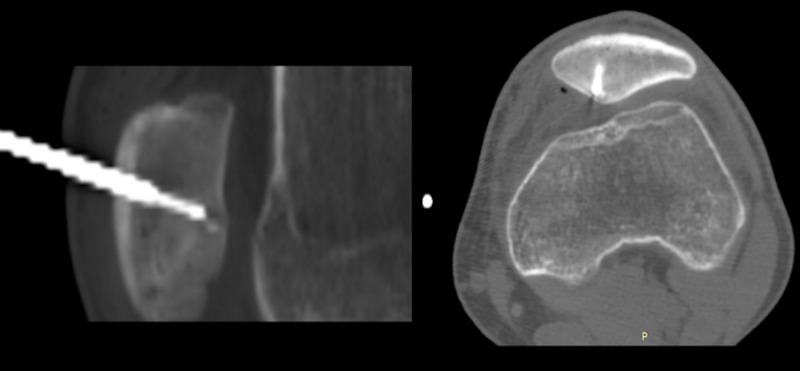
CT-guided RF ablation The needle tip (white), as it approaches the nidus RF: radiofrequency
